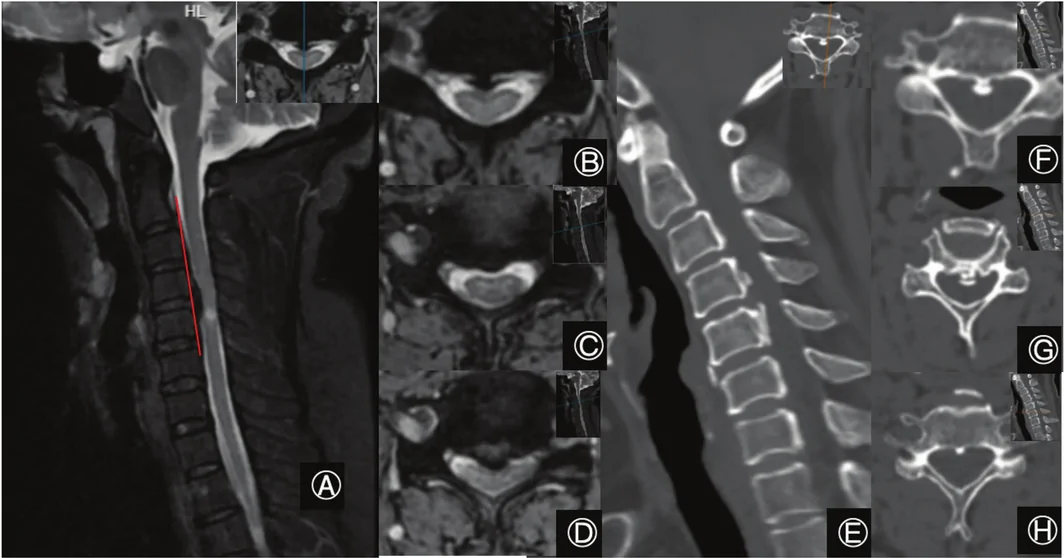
Case 2 Preoperative magnetic resonance imaging demonstrated: A negative K‐line and spinal cord compression at C3–C7 levels on coronal views.
